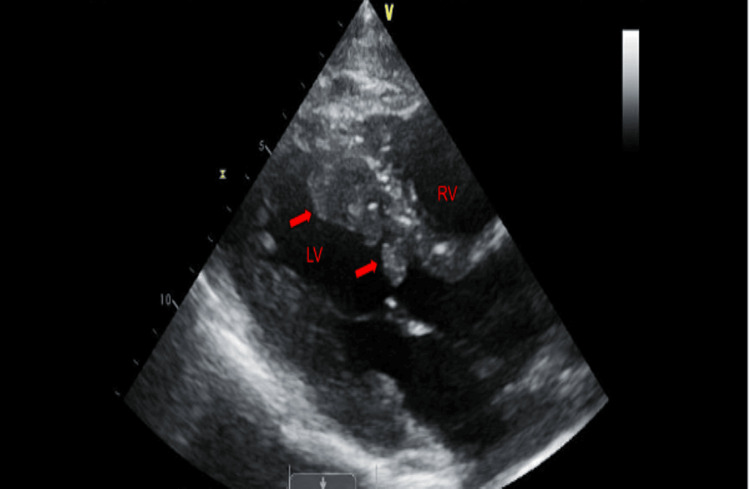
2D echocardiogram, parasternal view showing multiple masses in the left ventricular (LV) cavity. RV, Right ventricle
